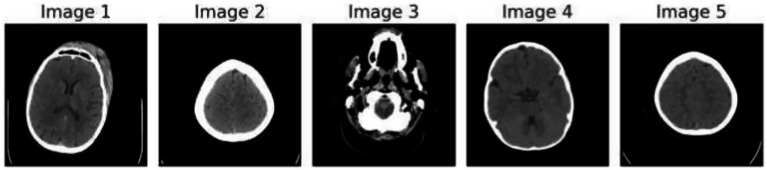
CT scan cross-sections showing brain structure and potential abnormalities.
